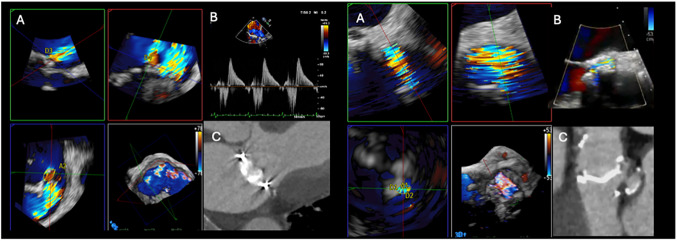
The TEE and CT images for assessing PVL grading. Left: Mitral PVL — (A) 3DVCA, (B) RUPV systolic flow reversal, (C) CT angiography. Right: Aortic PVL — (A) 3DVCA, (B) 2D long axis PVL flow, (C) CT angiography. TEE, transesophageal echocardiography; PVL, paravalvular leak; 3DVCA, three-dimensional vena contracta area; RUPV, right upper pulmonary vein
